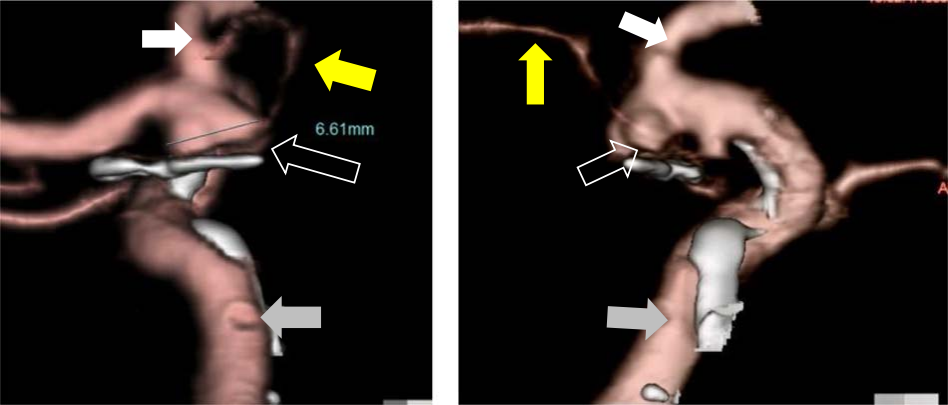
Preoperative CT angiography showed recurrent left ICA–AchA aneurysm and a surgical clip of the previous operation (open white arrow). The yellow arrow points to the AchA main trunk originating from ICA. The white and gray arrows indicate the anterior cerebral artery and ICA, respectively.
